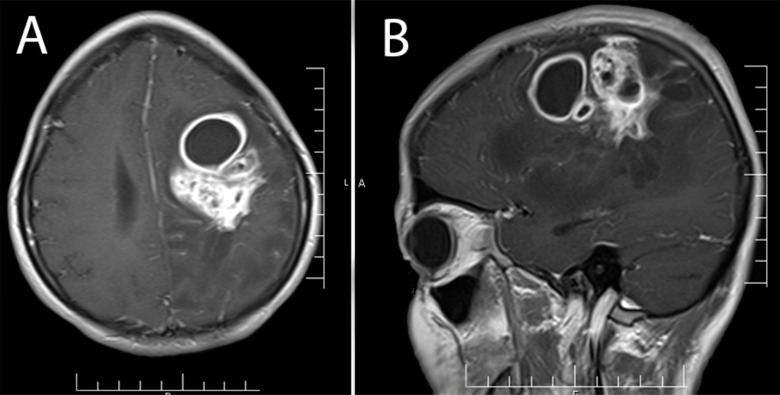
Axial (A) and sagittal (B) view of contrast-enhanced magnetic resonance imaging showed multiple ring enhancing lesions in the left frontoparietal
